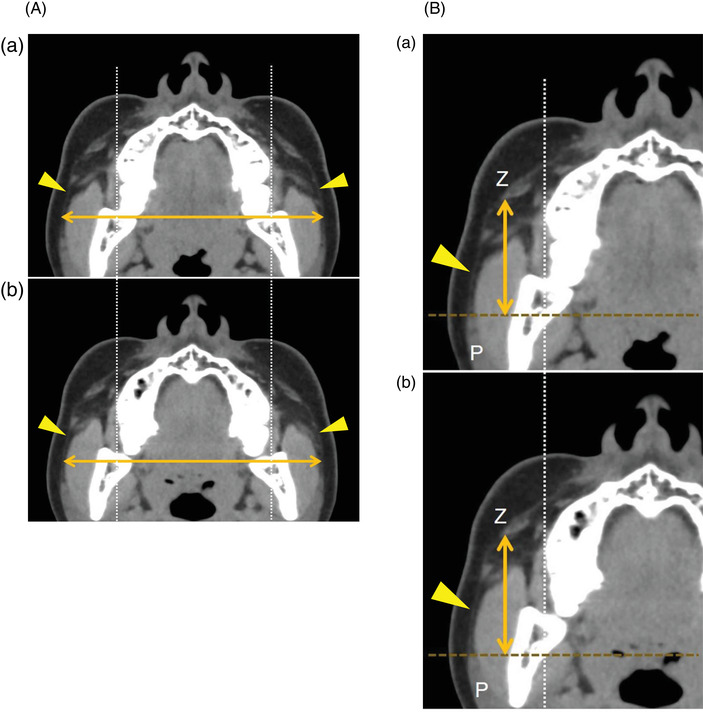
Superficial musculoaponeurotic system (SMAS) measurements. (A) Measurement of SMAS‐width. This computed tomography (CT) scan depicts the SMAS as a subcutaneous linear structure in the adipose tissue (yellow arrowheads). On a reconstructed axial CT image, the widest horizontal distance of the SMAS between the right and left margins of the SMAS was defined as the SMAS‐width. The SMAS‐width pre‐massage was measured. Subsequently, reconstructed axial post‐massage images corresponding to the same level as the pre‐massage image were created using the stationary facial bone as a basis for the measurements. The SMAS‐width post‐massage was then measured. Change rate of the SMAS‐width (%).  = (SMAS‐width post‐massage/SMAS‐width pre‐massage) × 100. (B) Measurement of SMAS‐height. On a reconstructed axial CT image, the highest vertical distance of the SMAS (yellow arrowhead) from the anterior edge of the parotid gland (P) to the back of the major zygomatic muscle (Z) was defined as the SMAS‐height. The SMAS‐height pre‐massage was measured, and the SMAS‐height post‐massage was measured on the reconstructed axial image. Change rate of the SMAS‐height (%). = (SMAS‐height post‐massage/SMAS‐height pre‐massage) × 100
